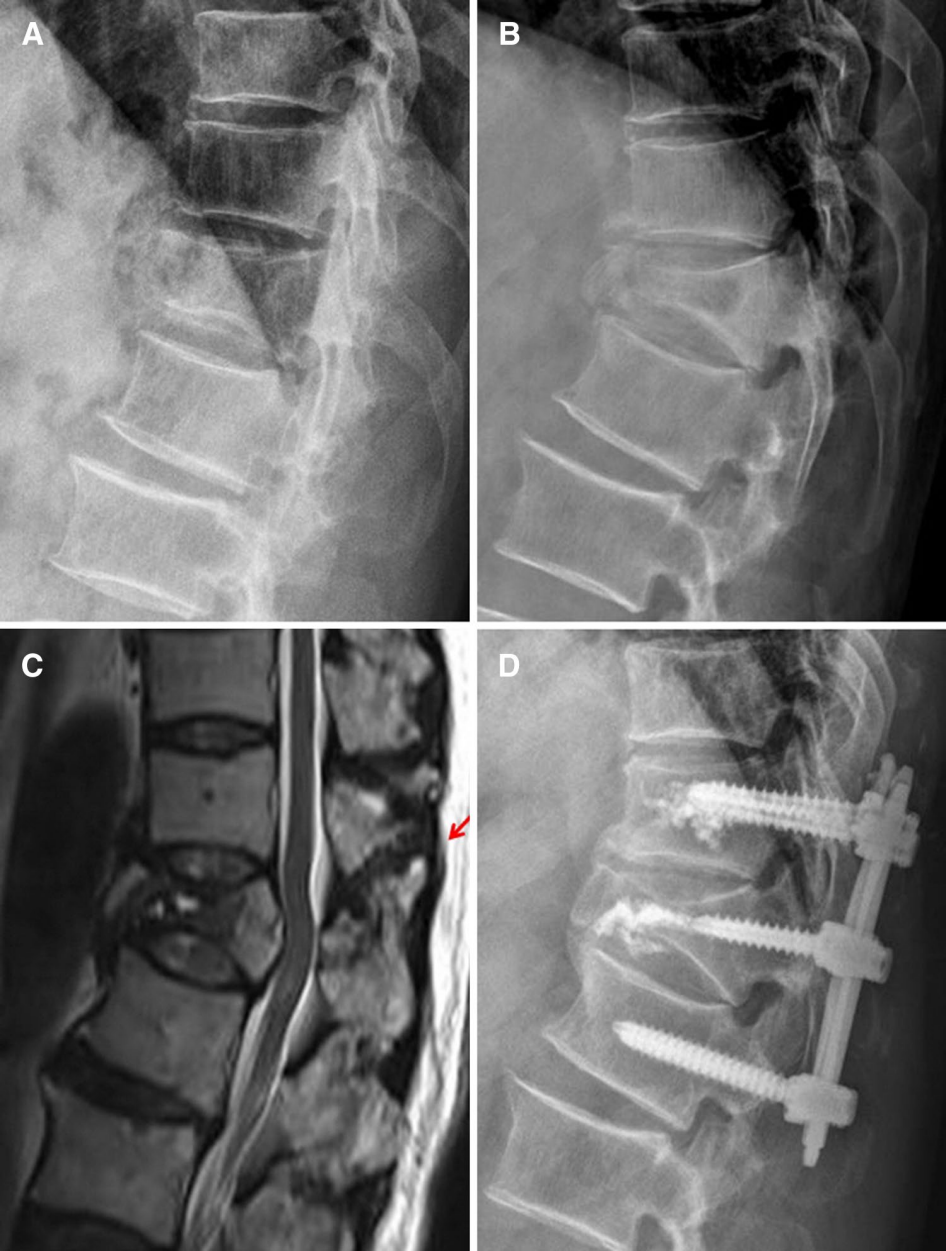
(A) A 64-year-old male patient was initially diagnosed with T12 stable burst fracture. (B) Kyphosis increased along with a collapse of the vertebral body after 2 weeks. (C) MRI showed further collapse of the vertebral body and occult injury of posterior ligamentous complex (red arrow). (d) At three weeks after the initial injury, patient underwent posterior fixation.
